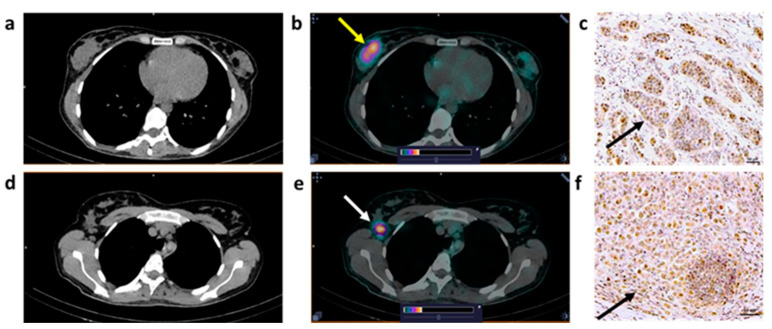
CT (a,d) and fused SPECT/CT (b,e) images of BCa patient (B2, invasive carcinoma) 2 h after injection of [^99m^Tc]Tc-maSSS-PEG_2_-RM26. A focus of increased [^99m^Tc]Tc-maSSS-PEG_2_-RM26 uptake (SUVmax = 1.75) is visualized in the right breast ((b), yellow arrow). An enlarged (up to 1.3 cm) right axillary node with elevated [^99m^Tc]Tc-maSSS-PEG_2_-RM26 uptake (SUVmax = 1.8) is visualized ((e), white arrow).The upper setting of the scale window (24% of the maximum number) was adjusted to visualize the lesion. Light (1+) GRPR expression and moderate (2+) expression (black arrow) in carcinoma cells were detected by IHC analysis in primary BCa tumor (c) and in LN (f). Magnification 40×.
